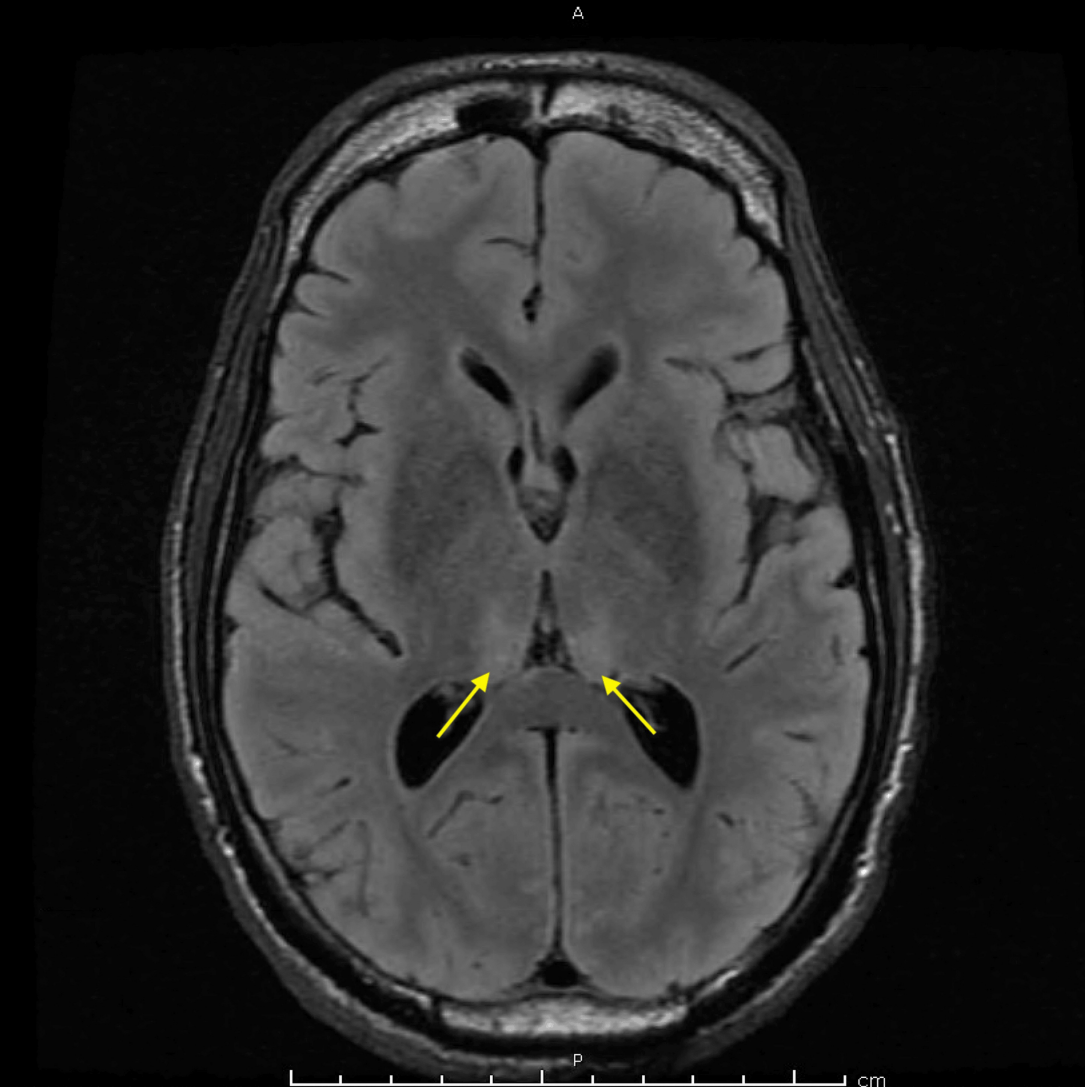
MRI fluid-attenuated inversion recovery (FLAIR) of the brain revealed acute bilateral paramedian thalamic infarcts indicated by the yellow arrows.
